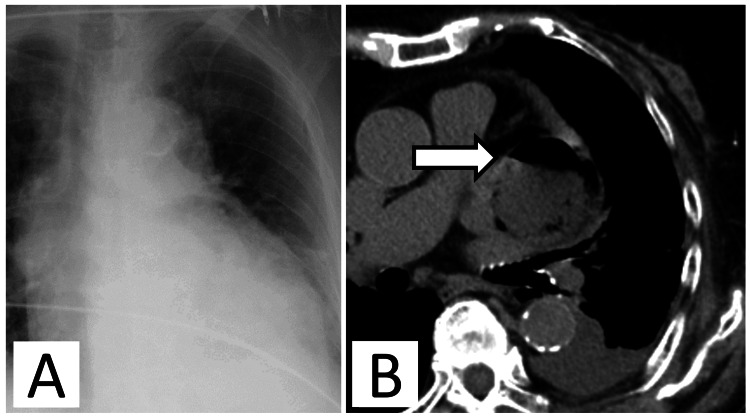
Imaging findings on the day of admission. (A) Chest x-ray showing cardiomegaly. (B) Axial chest computed tomography (CT) revealing fluid accumulation in the pericardial space; air is observed inside the liquid, and an air-fluid level is observed (white arrow).
